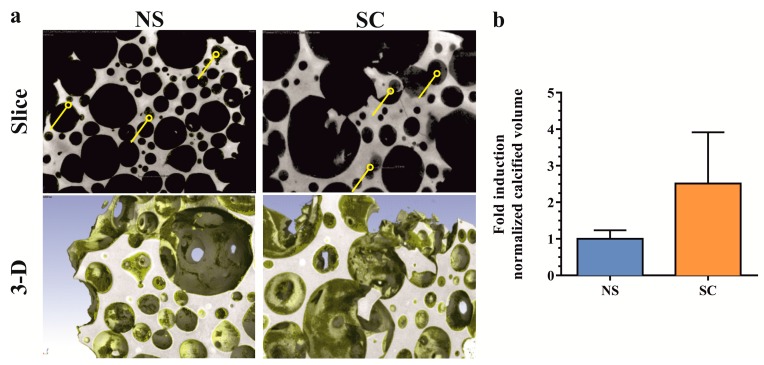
Quantification of de novo calcified tissue by micro-computed tomography. (a) Evidence of de novo calcified tissue formed in scaffold pores (arrowheads, upper panels). Three-dimensional visualization of ceramic (grey) and de novo calcified tissue (yellow) in explanted scaffold (lower panels). (b) Quantification of calcified tissue volume normalized by scaffold pore volume. Values are expressed as fold induction over non-sclerotic samples (means + SEM, *n* = 3 donors, duplicate implantations). NS: non-sclerotic, SC: sclerotic.
